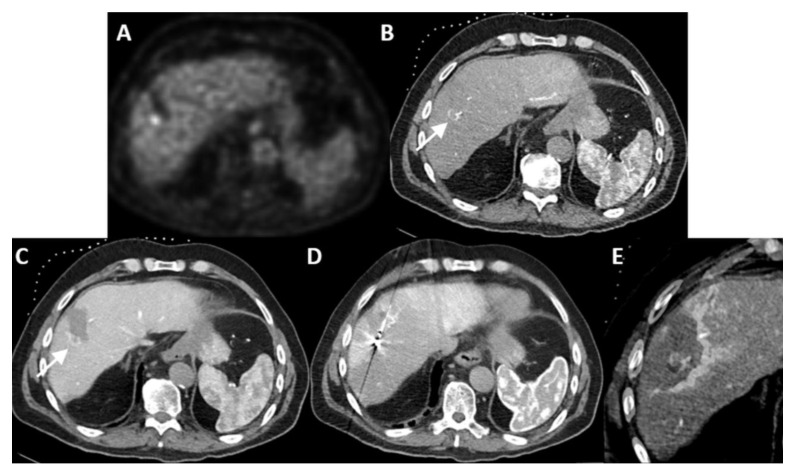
Differentiation between residual or recurring tumor tissue and non-enhancing scar tissue. Follow-up ^18^F-FDG PET-CT showed focal FDG uptake at the edge of the ablation zone in segment VIII (A). Catheterization of the coeliac trunk and intra-arterial administration of 40 mL Xenetix 300^®^ (1:1 mixed bolus of saline and contrast agent; Iobitridol 300 mg Iodine/mL, Guerbet, Villepinte, France) showed a typical incomplete enhancing ring (arrow), identified at the interface with the post-ablation scar tissue ((B), arterial phase at 7 s; (C), portal venous phase at 22 s). Intraprocedural verification of the microwave needle (Emprint™ Microwave Ablation System, Medtronic-Covidien, Boulder, CO, USA) (D). Overlay of pre- and postprocedural CT images (image fusion) showed complete coverage of the tumor by the ablation zone (E).
